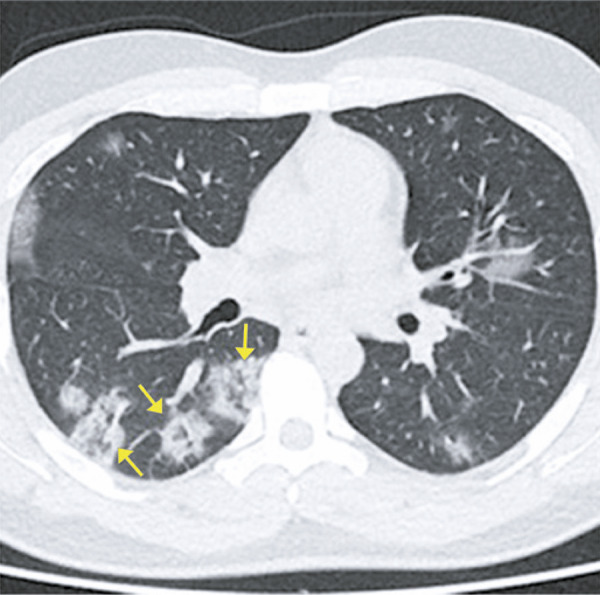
A 38-year-old patient with COVID-19, presenting fever, dry cough, malaise and headache for six days. Computed tomography demonstrating reversed halo sign in the lower lobes
